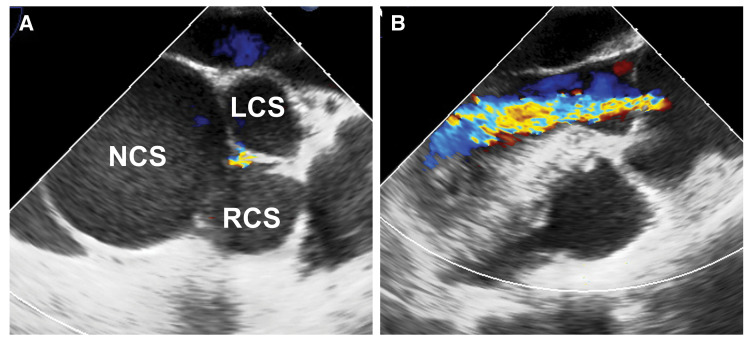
Preoperative transesophageal echocardiographic images. (A) Short-axis view of the aortic valve showing that the NCS is larger than the LCS and RCS. (B) Color Doppler long-axis view showing central moderate AR. AR, aortic regurgitation; LCS, left-coronary sinus; NCS, noncoronary sinus; RCS, right-coronary sinus
